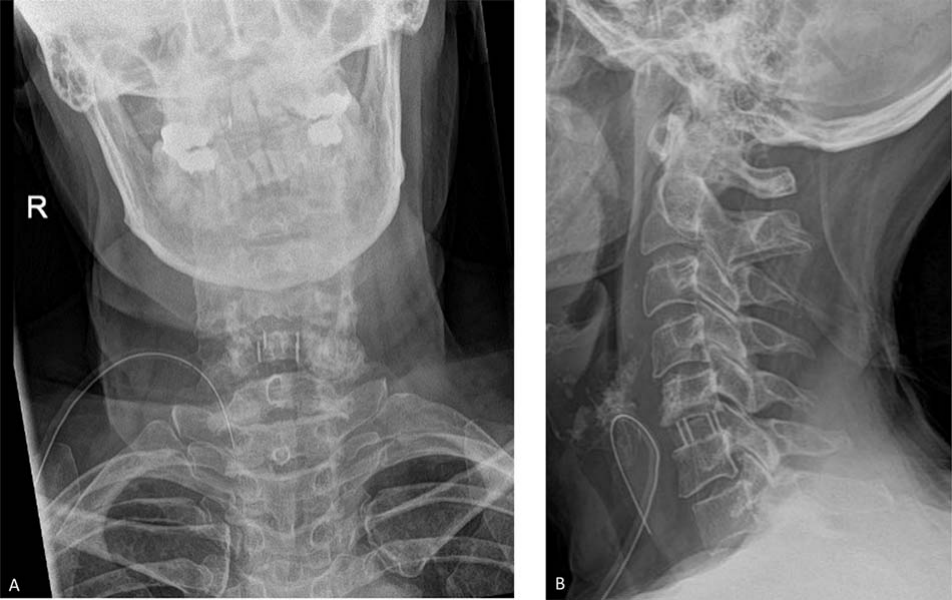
Post-operative radiographs. A. Anteroposterior view. B. Lateral view. The C5/C6 disc was identified using X-ray guidance and the vertebral bodies were distracted using Caspar pins. The endplates were prepared with a curette and the discectomy was performed. The posterior osteophytes were thinned with a matchstick drill and the posterior longitudinal ligament and posterior osteophytes were removed with Kerrison rongeurs.
